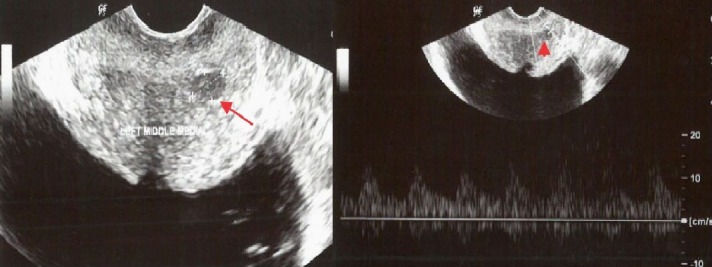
TRUS and power Doppler in a 54-year-old male The images show a small, well-defined, oval-shaped hypoechoic nodule (arrow) in the peripheral zone. The color Doppler image shows focal increased vascularity in the region of the nodule. DRE was negative and PSA was slightly increased.
